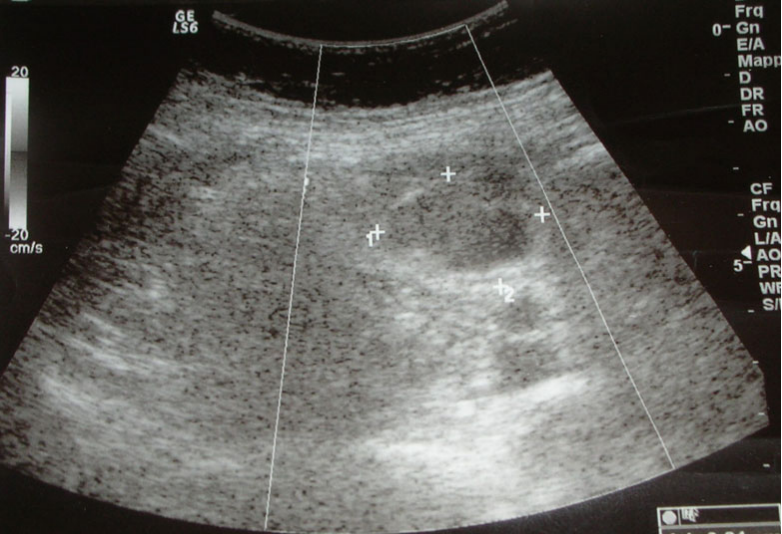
Ultrasonography showing a 3.2 cm pre-aortic mass.
